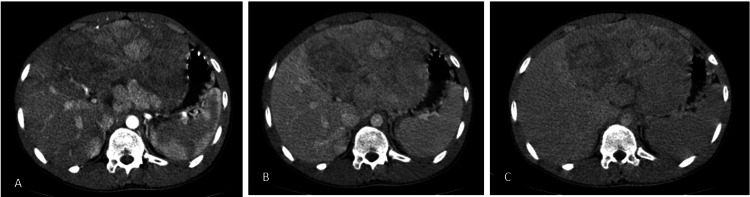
Contrast-enhanced CT abdomen CT: Computed tomography (A) Arterial phase, (B) portovenous phase, and (C) delayed phase images showing heterogeneously enhancing multifocal intraparenchymal and subcapsular lesions involving segments IVA, II, III and IVB of the left lobe of the liver with persistent enhancement and no evidence of washout
